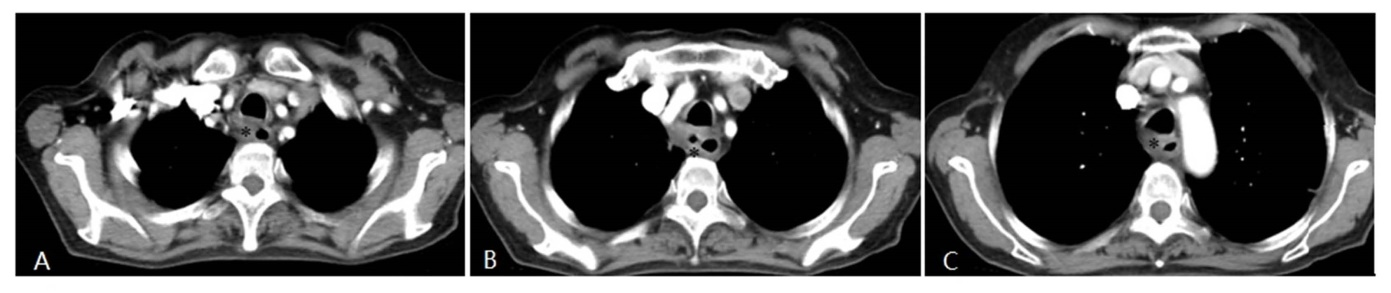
Four weeks after interventional therapy, a chest CT scan shows that the lesion has significantly reduced, tracheal lumen is normal, and the arterial phase enhancement is not apparent (*) (A, B, C).
